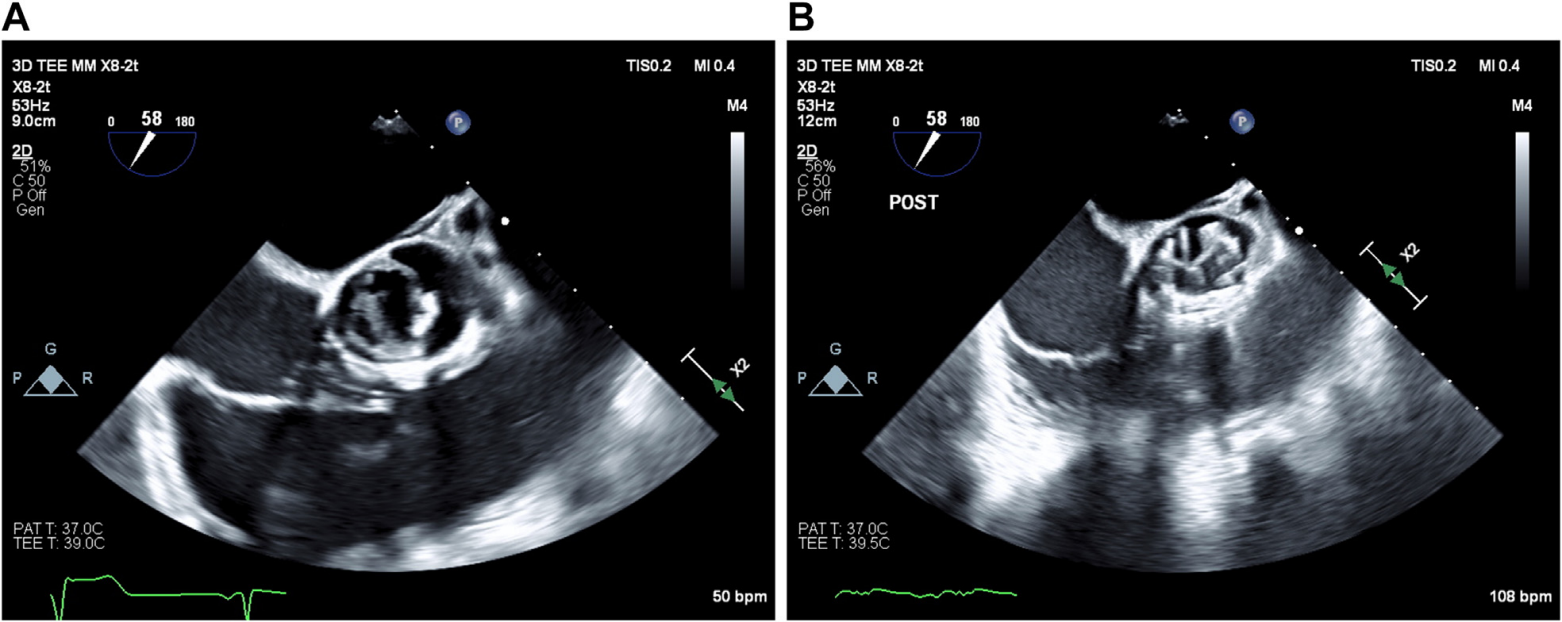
Transesophageal Echocardiogram of the Aortic Valve (A) Before transcatheter aortic valve replacement. (B) Post transcatheter aortic valve replacement. bpm = beats/min; G = general; P = penetration; PAT = patient; R = resolution; T = temperature; TEE = transesophageal echocardiogram; 2D = 2-dimensional.
